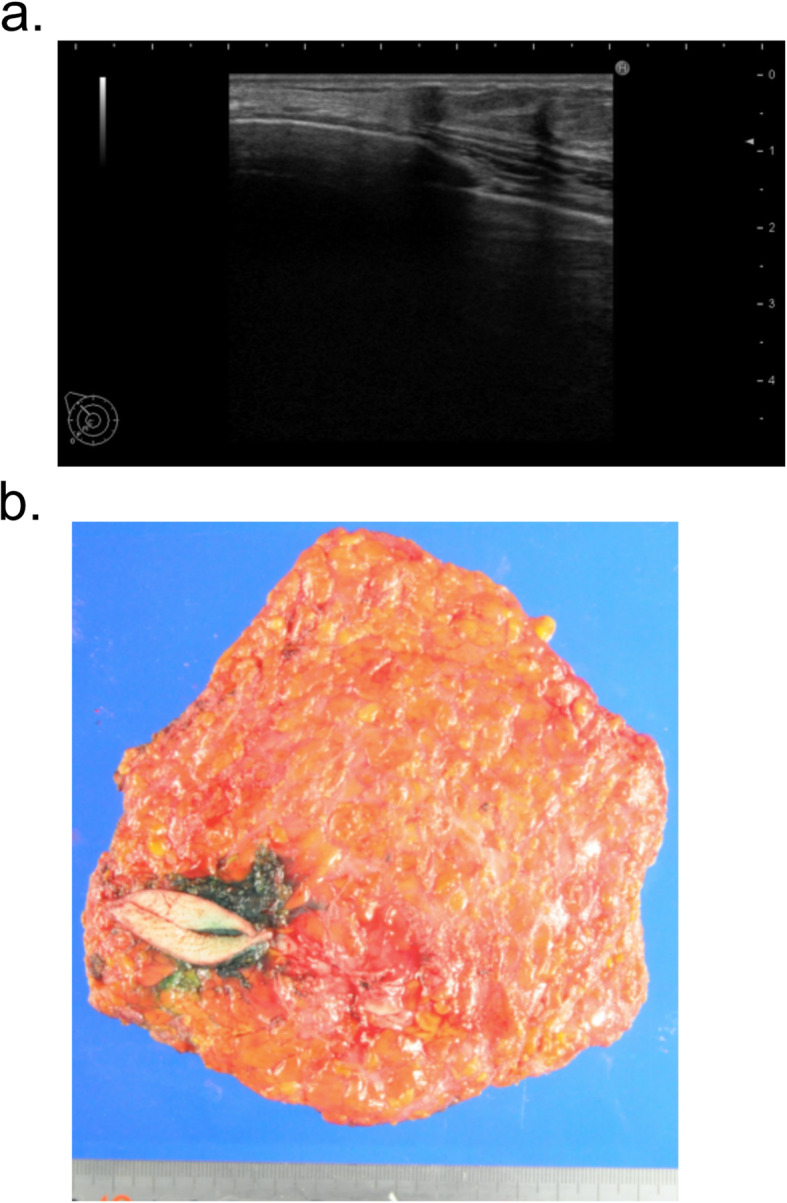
A case in which the tumor-to-dermis distance was less than 2 mm underwent resection of the skin immediately overlying the tumor. a Ultrasonography revealed that tumor-to-dermis distance was less than 2 mm. b Resection of the skin just above the tumor was performed
